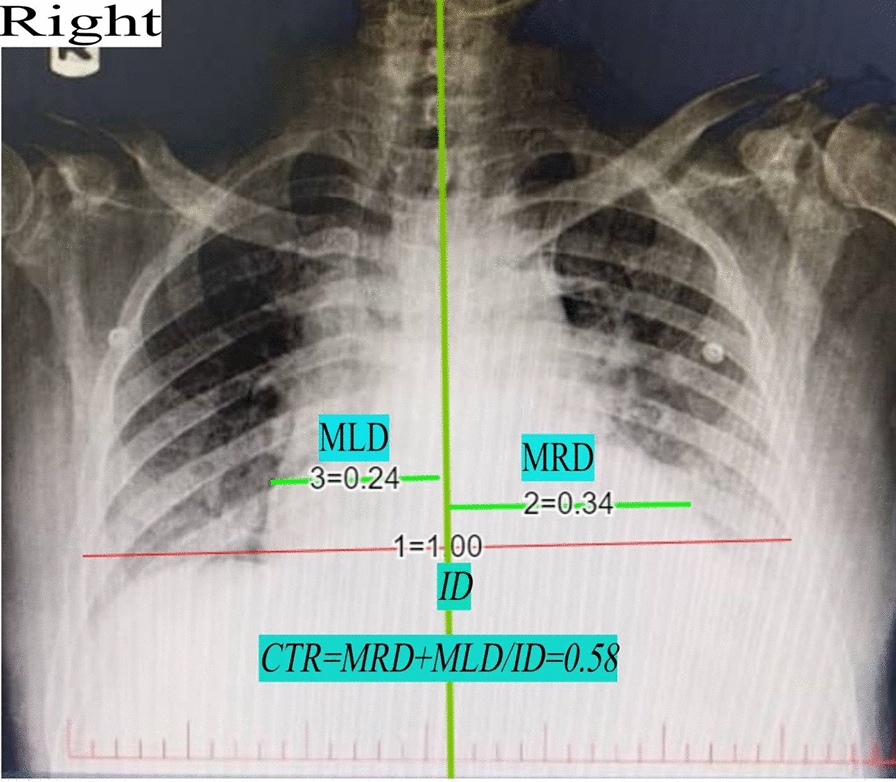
Chest X-ray of the patient with cardiac tamponade showing an enlarged, water bottle-shaped cardiac silhouette, indicative of significant pericardial effusion. *MLD* midline-left diameter, *MRD* midline-right diameter, *ID* internal diameter, *CTR* cardiothoracic ratio
